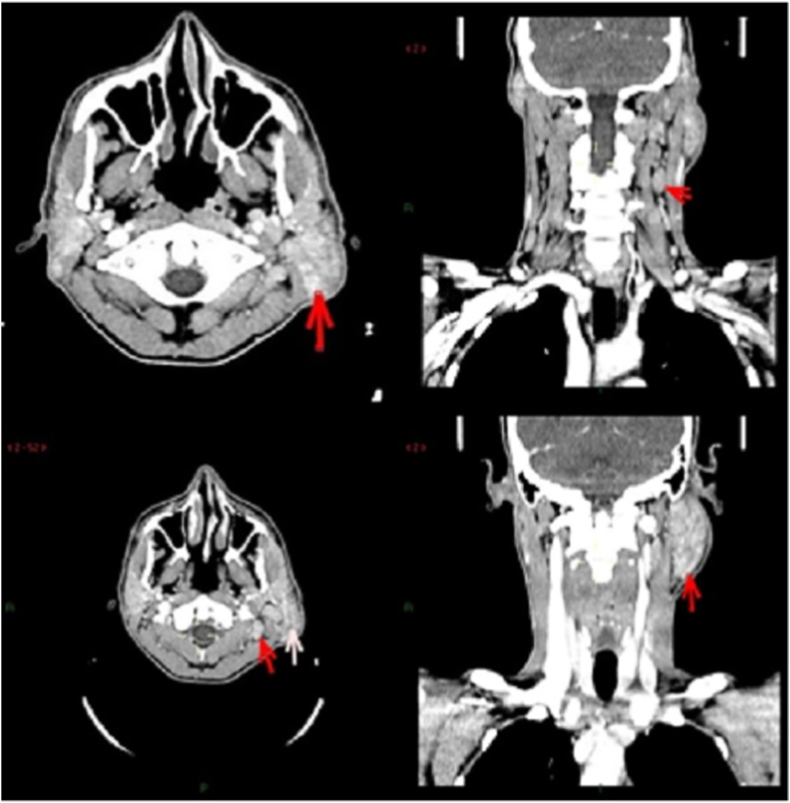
CT scan of patient pre-operatively.
